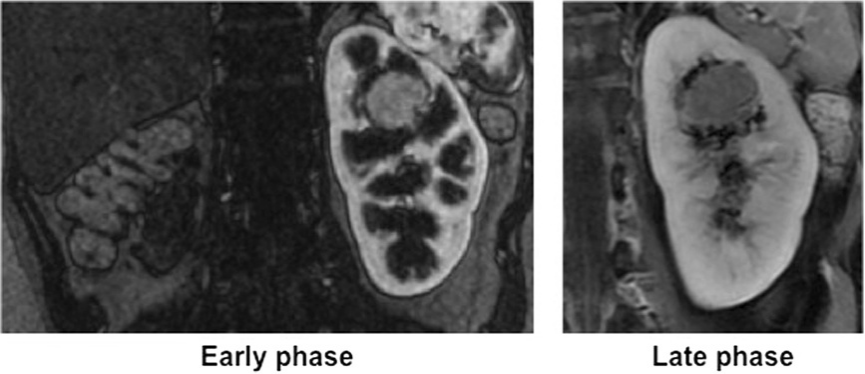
Contrast-enhanced coronal T1-weighted imaging with fat saturation (left: arterial phase; right: late phase) reveals a hypervascular solitary solid mass in the upper half of the left (functional) kidney.
